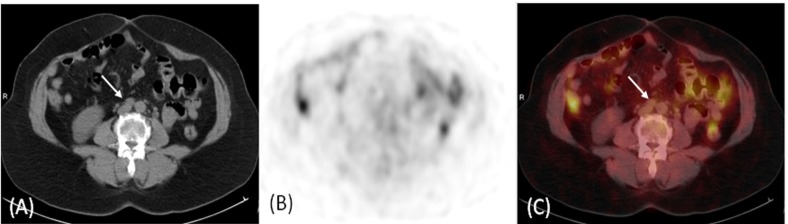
A 55 year-old female patient with cervical cancer underwent ^18^F -FDG PET/CT for staging. (A) Transaxial CT scan images demonstrated suspicious aorto-caval lymph nodes (arrow). Corresponding transaxial ^18^F -FDG PET (B) and transaxial fused ^18^F -FDG PET/CT (C) images didn’t demonstrate increase in FDG uptake in these lymph nodes (arrow). Patient received radical treatment to the pelvic diseases and follow up didn’t demonstrate any progression in these retroperitoneal lymph nodes
